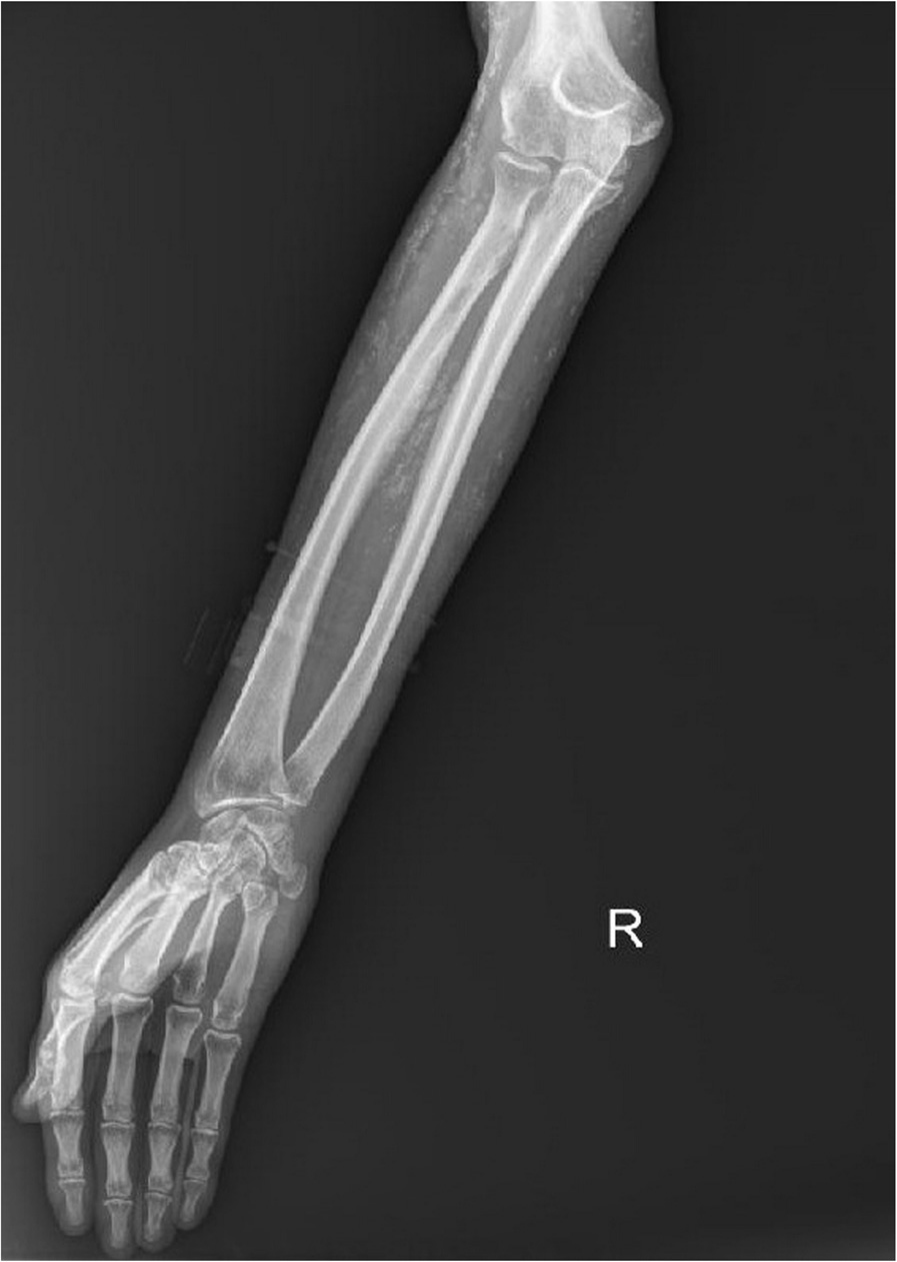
Plain radiographs showing soft-tissue calcification along right arm.
